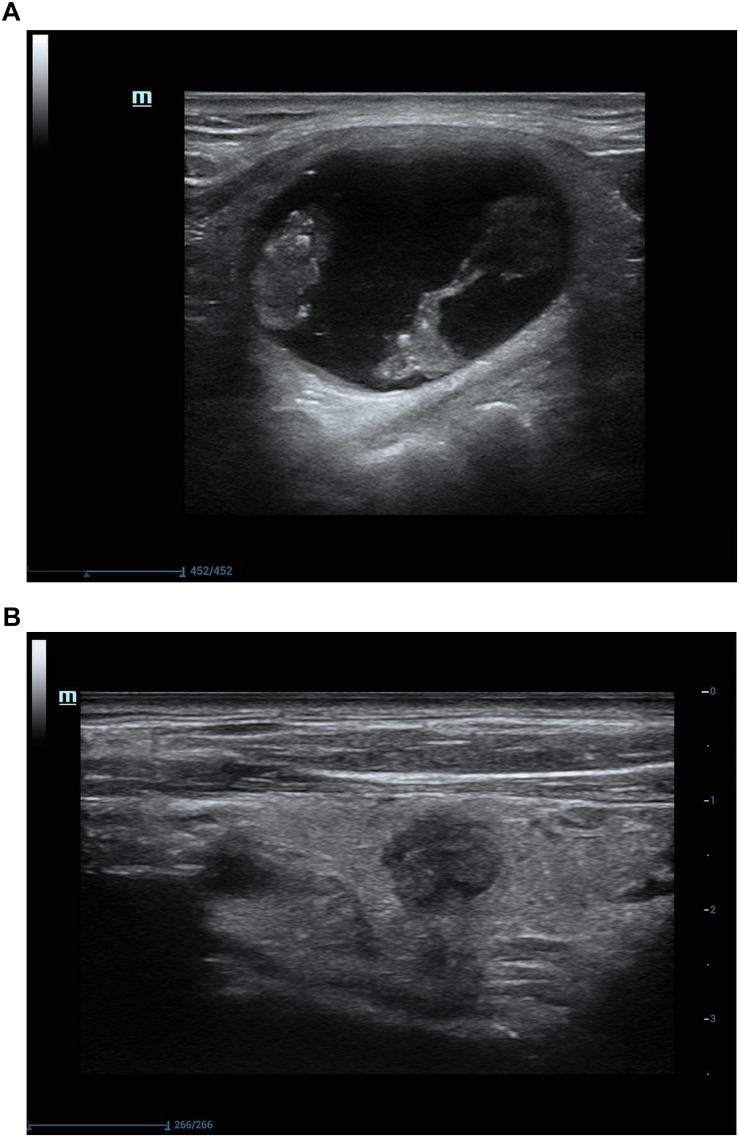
Ultrasonograpic image of a 62 years old male before and after treatment.(A) A predominantly cystic nodule before USG-LIA.(B) 12 months after USG-LIA.
